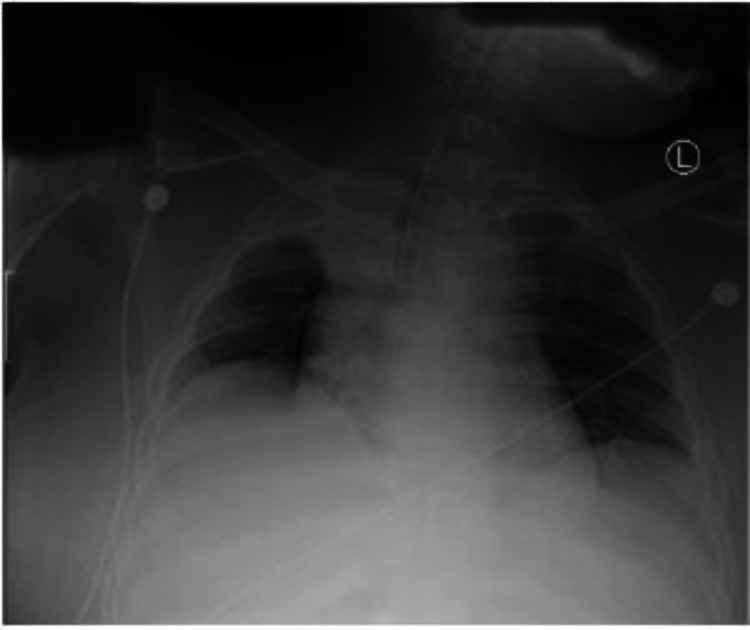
X-ray image - post foreign body removal
